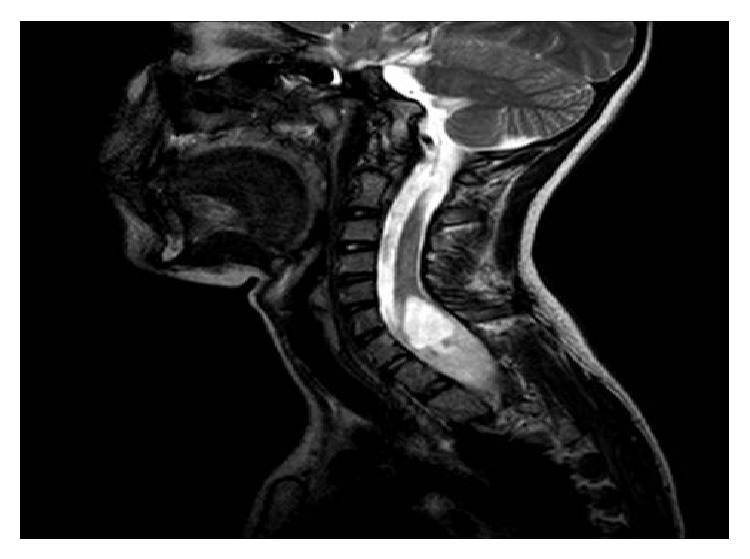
Sagittal turbo spin-echo (TSE) T2-weighted MRI of cervical spine demonstrates a large expansile intra-axial mass of the spinal cord. The upper cystic part and the lower solid parts of the mass are hyperintense.
